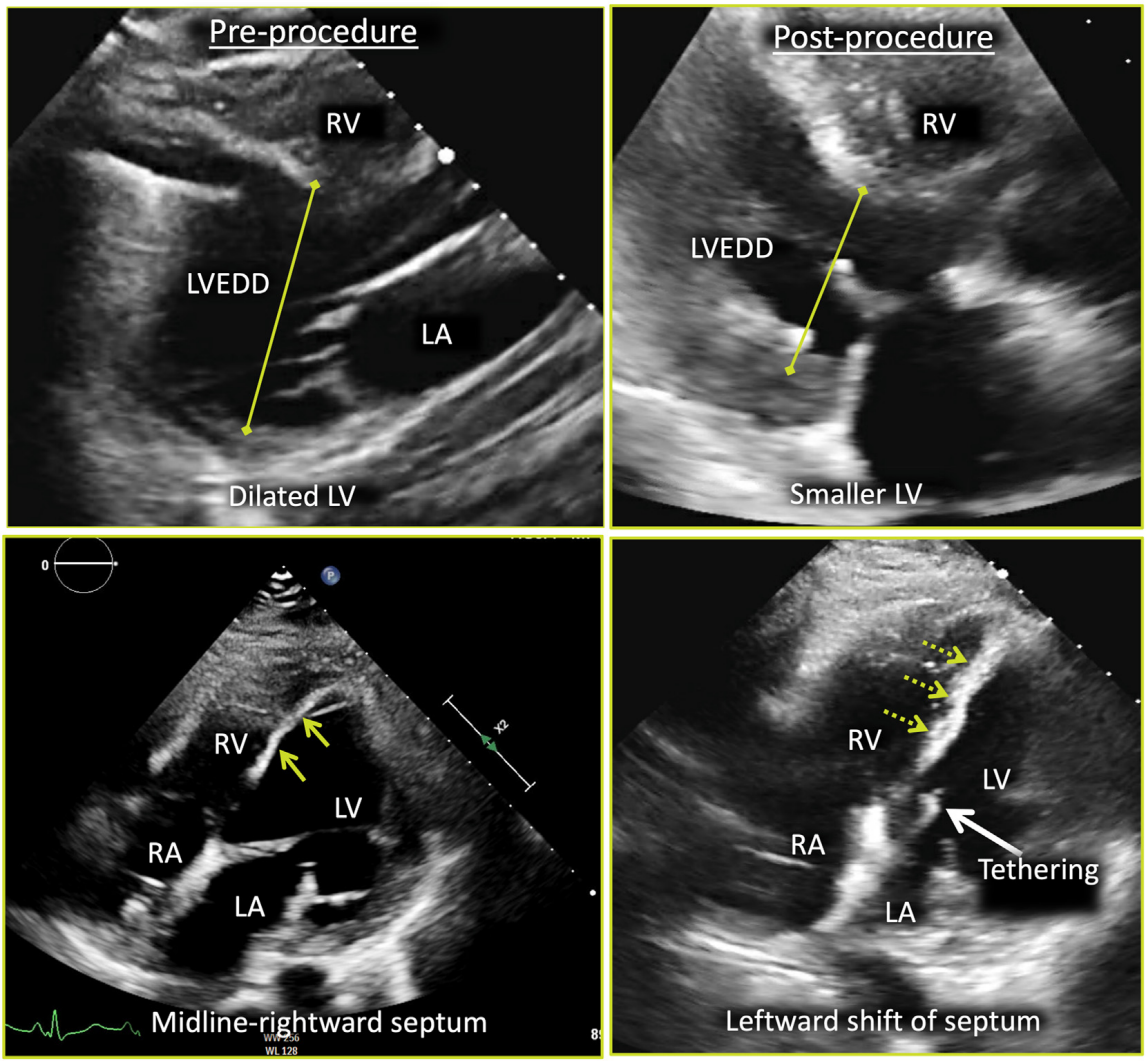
Two-dimensional TTE preprocedure *(left)* and postprocedure after drainage of the MFC *(right)*, parasternal long-axis *(top)* and right heart–focused apical four-chamber *(bottom)* views, demonstrates the reduction in left ventricular cavity, dilation in right ventricular cavity, leftward shift of the IVS *(yellow arrows)*, and tethering of the mitral valve leaflets *(white arrow)*. *LA*, Left atrium; *LV*, left ventricle; *LVEDD*, left ventricular end-diastolic diameter; *RA*, right atrium.
